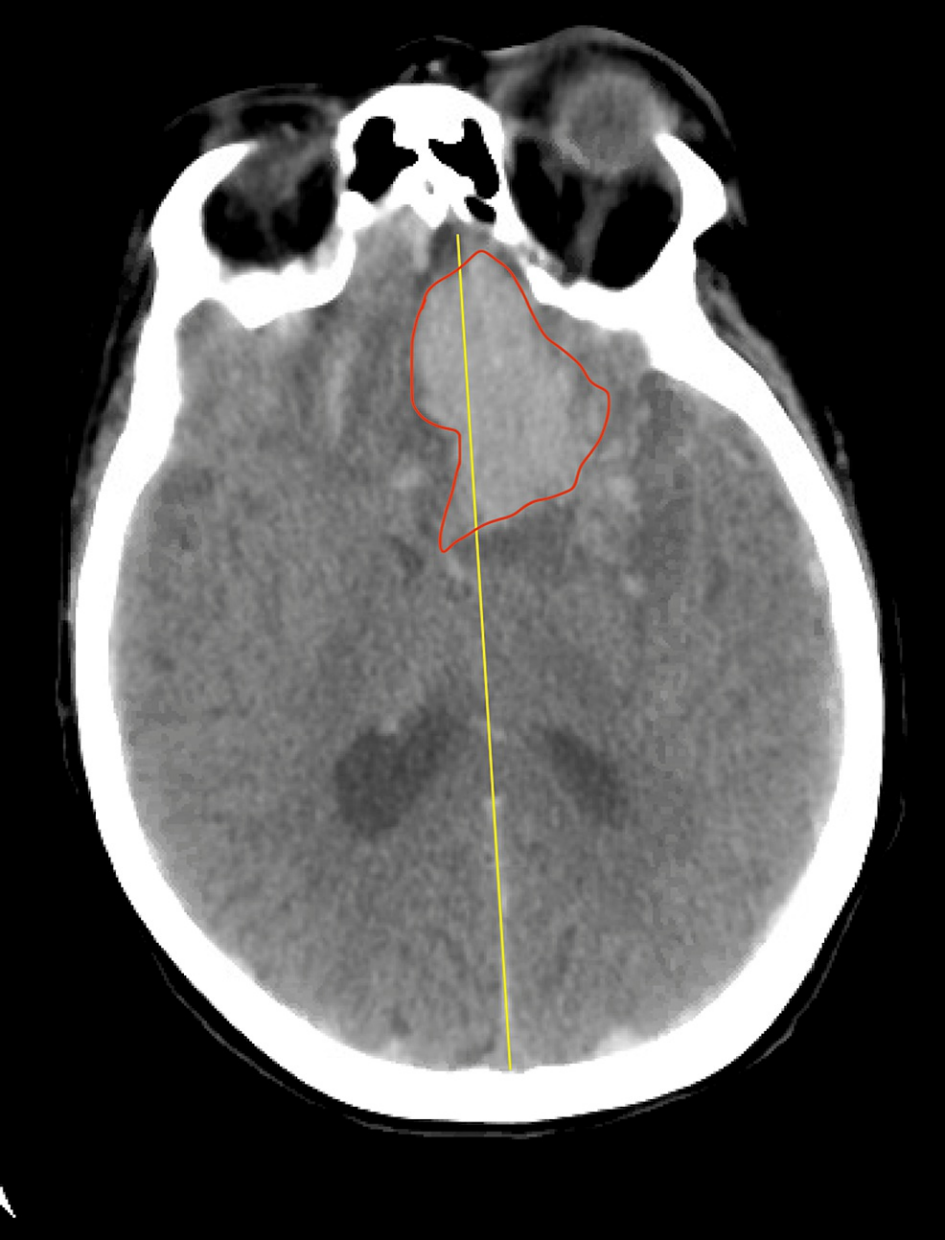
Patient's non-contrast CT scan The CT scan image is demonstrating a large intraparenchymal hematoma (red outline) and  shift away from the midline (yellow line)
